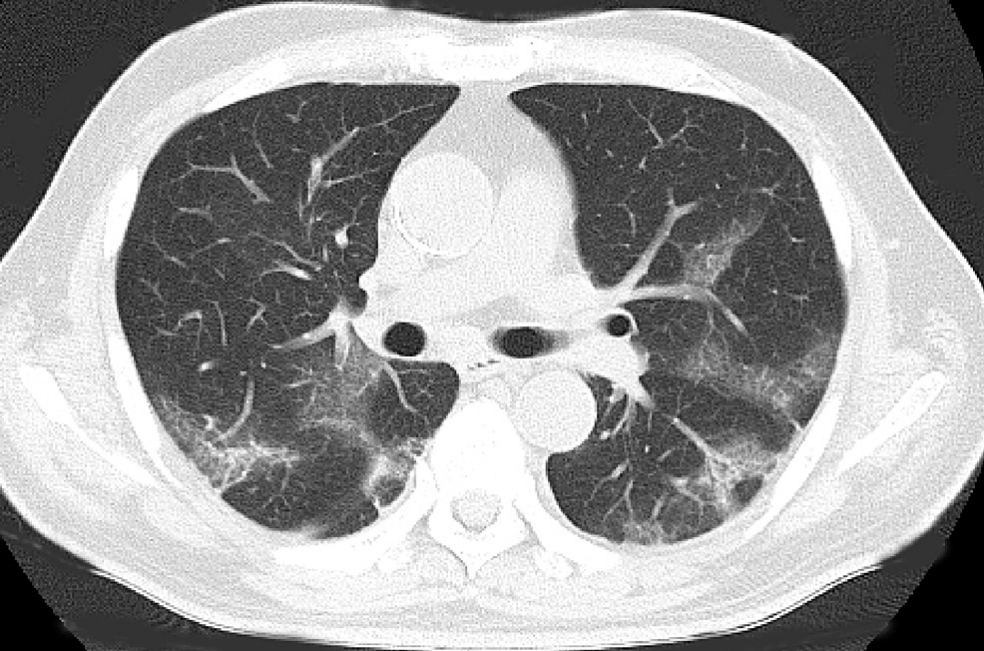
Chest computed tomography showing subpleural ground-glass shadows in the bilateral lower lobes. Typical image of acute interstitial pneumonia as coronavirus disease 2019.
